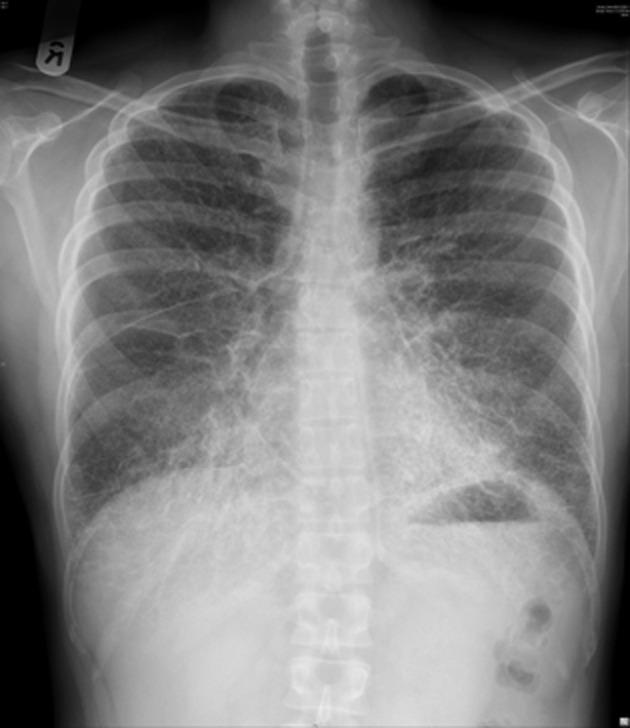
Plain chest radiograph showing a typical “sandstorm” appearance of pulmonary alveolar microlithiasis.
